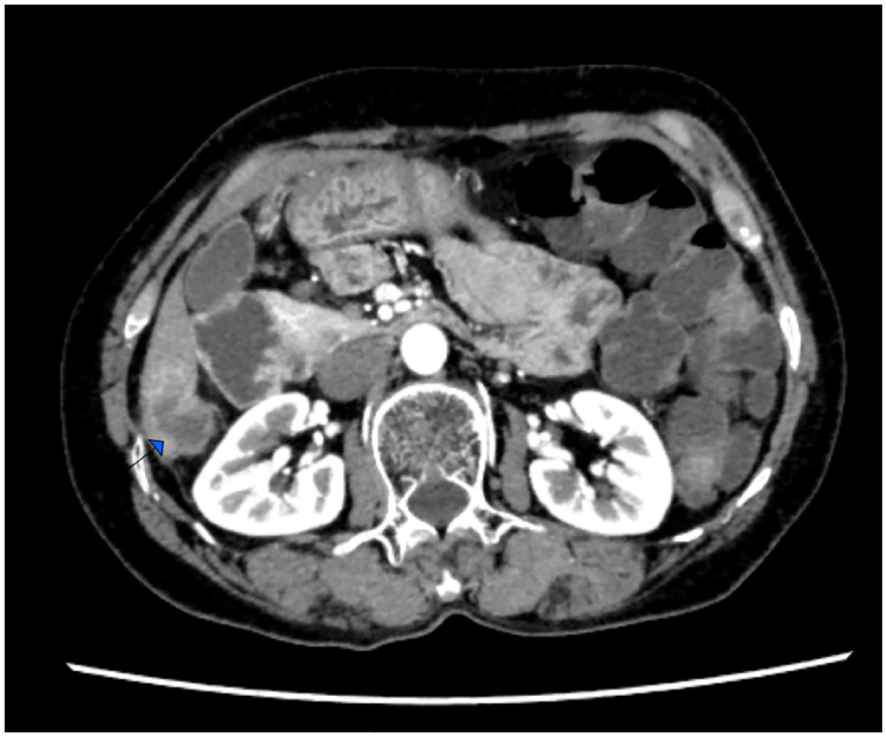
Computedtomography (CT) scan, a solitary nodule was detected in the liver, indicating a metastatic lesion.
